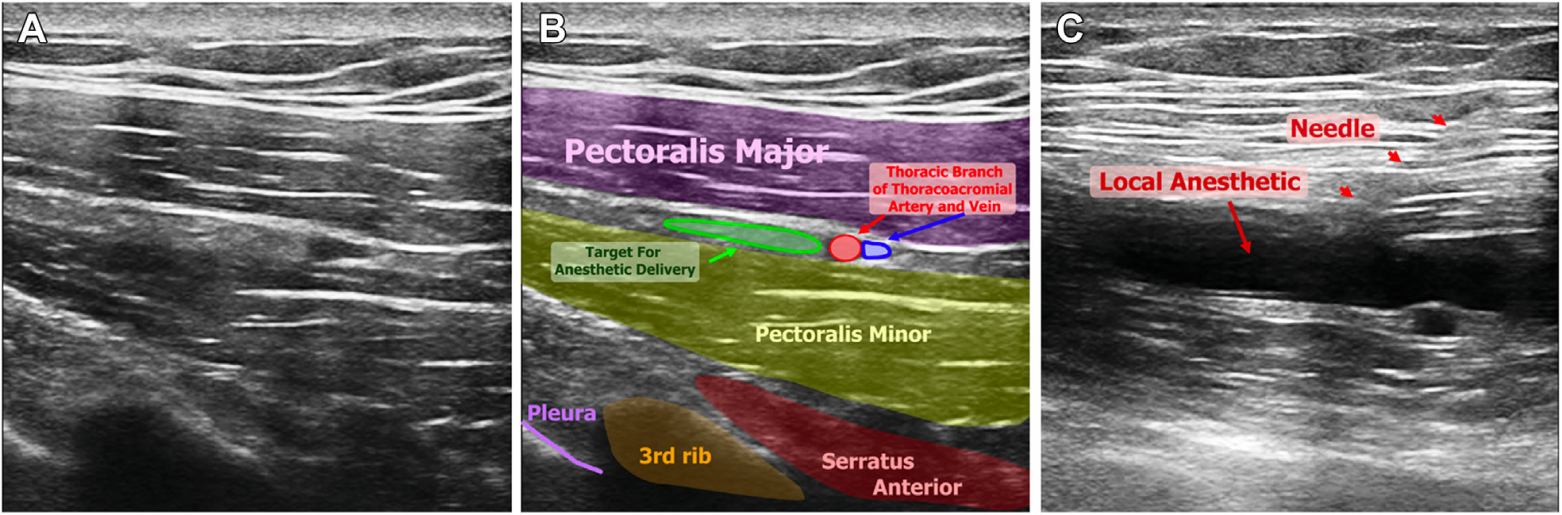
Ultrasound-Guided SCN Block (A) The ultrasound probe is placed on the lateral aspect of the neck in the sagittal plane posterior to the sternocleidomastoid muscle. The vertical landmark is the crossing of the external jugular vein with the posterior border of the sternocleidomastoid muscle at approximately one-third of the neck height. (B) Same image with a labeled overlay. (C) Approach to delivering local anesthetic in the area of the SCN taking care to keep the needle tip above the prevertebral fascia to avoid anesthetizing the brachial plexus. SCN = supraclavicular nerve.
